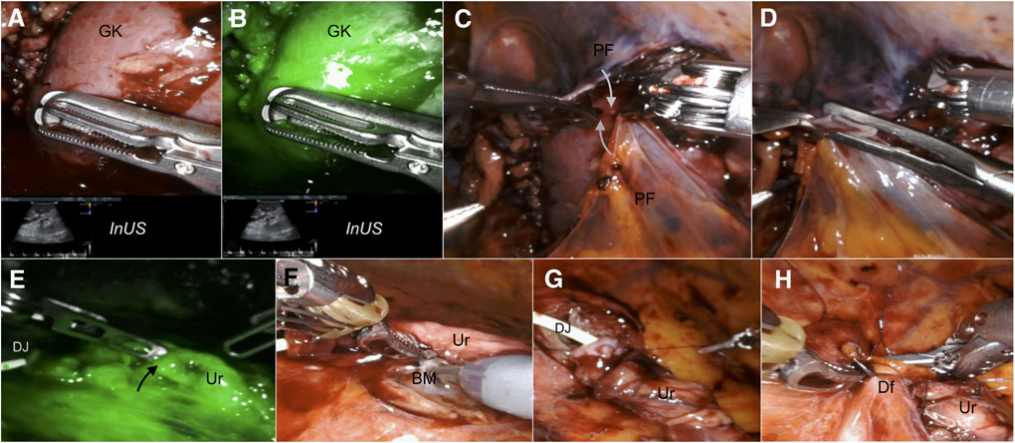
Intraoperative snapshots showing graft reperfusion and the key steps of ureterovesical anastomosis in our case. (A, B, E). After venous injection of 0.3 mg/kg of indocyanine green, Firefly^®^ fluorescence imaging technology and InUS were used to check graft reperfusion and integrity of ureteral vascularization (*black arrow*). (C, D). Closure of the extraperitoneal pouch reapproximating the two previously prepared PF by using hem-o-Lok clips (*white arrows*). (F–H). Key steps of ureteroneocystostomy using a modified Lich-Gregoir technique. After spatulation, the Ur is anastomosed to the bladder mucosa in a continuous fashion over the preplaced DJ; then, the detrusor muscle was closed with a running suture creating an antirefluxing mechanism. BM, bladder mucosa; Df, detrusor flap; InUS, intraoperative duplex ultrasound; PF, peritoneal flaps; Ur, ureter.
